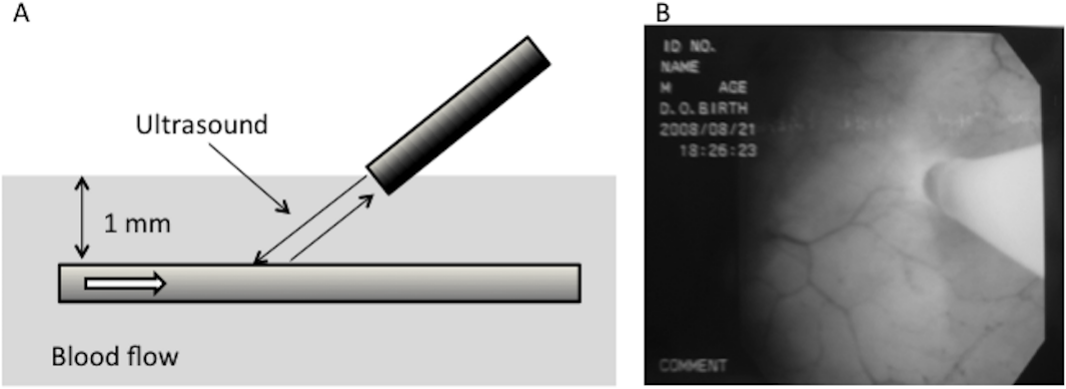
Laser Doppler flowmeter (LDF; OMEGA FLOW FLO-C1 BV, OMEGAWAVE, Tokyo, Japan). 1A: LDF employs a beam of low intensity monochromatic infrared light emitted from a laser diode within the flowmeter to measure the real-time micro-perfusion rate at tissue depths of 1 mm. B: The probe is placed a few mm away from the bladder mucosa while the bladder is filled with 150 ml saline at room temperature.
